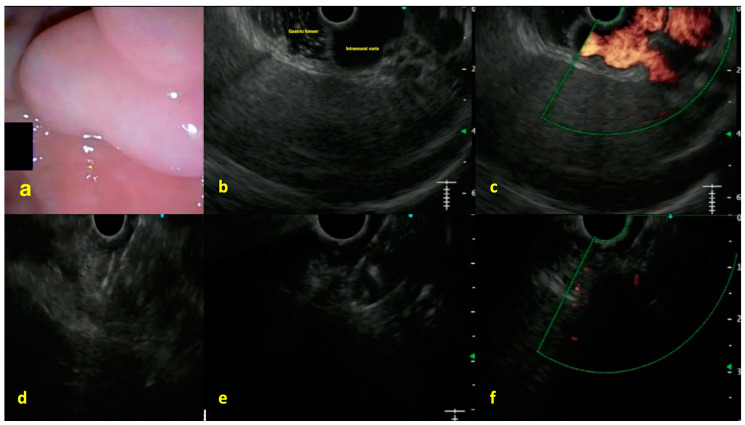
EUS-guided angioembolization of the gastric fundal varices (a) endoscopic view of the fundus reveals large varix (GOV2F2); (b) linear EUS examination was performed for variceal assessment by instillation of water in the fundus of the stomach and it revealed a large intramural varix (submucosal component); (c) Doppler assessment using EUS positive flow in varix; (d) varix was punctured using a 19-G EUS-FNA needle and Nester coils were deployed under EUS vision; (e) after the varix was packed with the sufficient number of coils, cyanoacrylate glue was injected under EUS-vision leading to the formation of the coil-glue cast; and (f) confirmation of complete variceal obliteration using color Doppler assessment (no positive flow noted) (permission has been taken from Dr. Jayanta Samanta for the publication of this image). The copyright of the image belongs to the authors.
